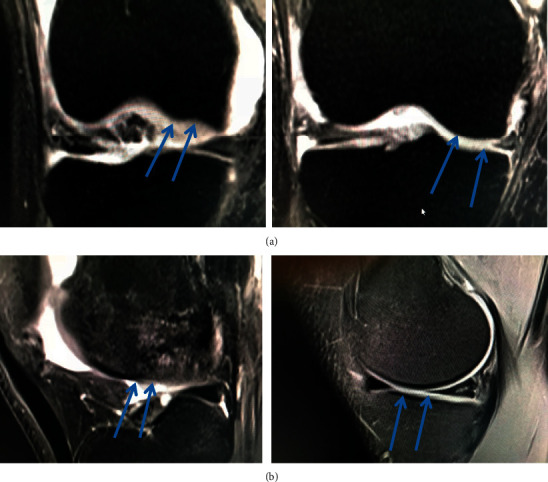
(a) AP view of the MRI of the knee joint showing depression in the femoral condyle (blue arrows) due to the damaged cartilage which completely recovered post injection of ABMDC. (b) Lateral view of the MRI of knee joint with erosion of the cartilage of the femoral condyle and post-injection regeneration of the damaged cartilage (blue arrows).
